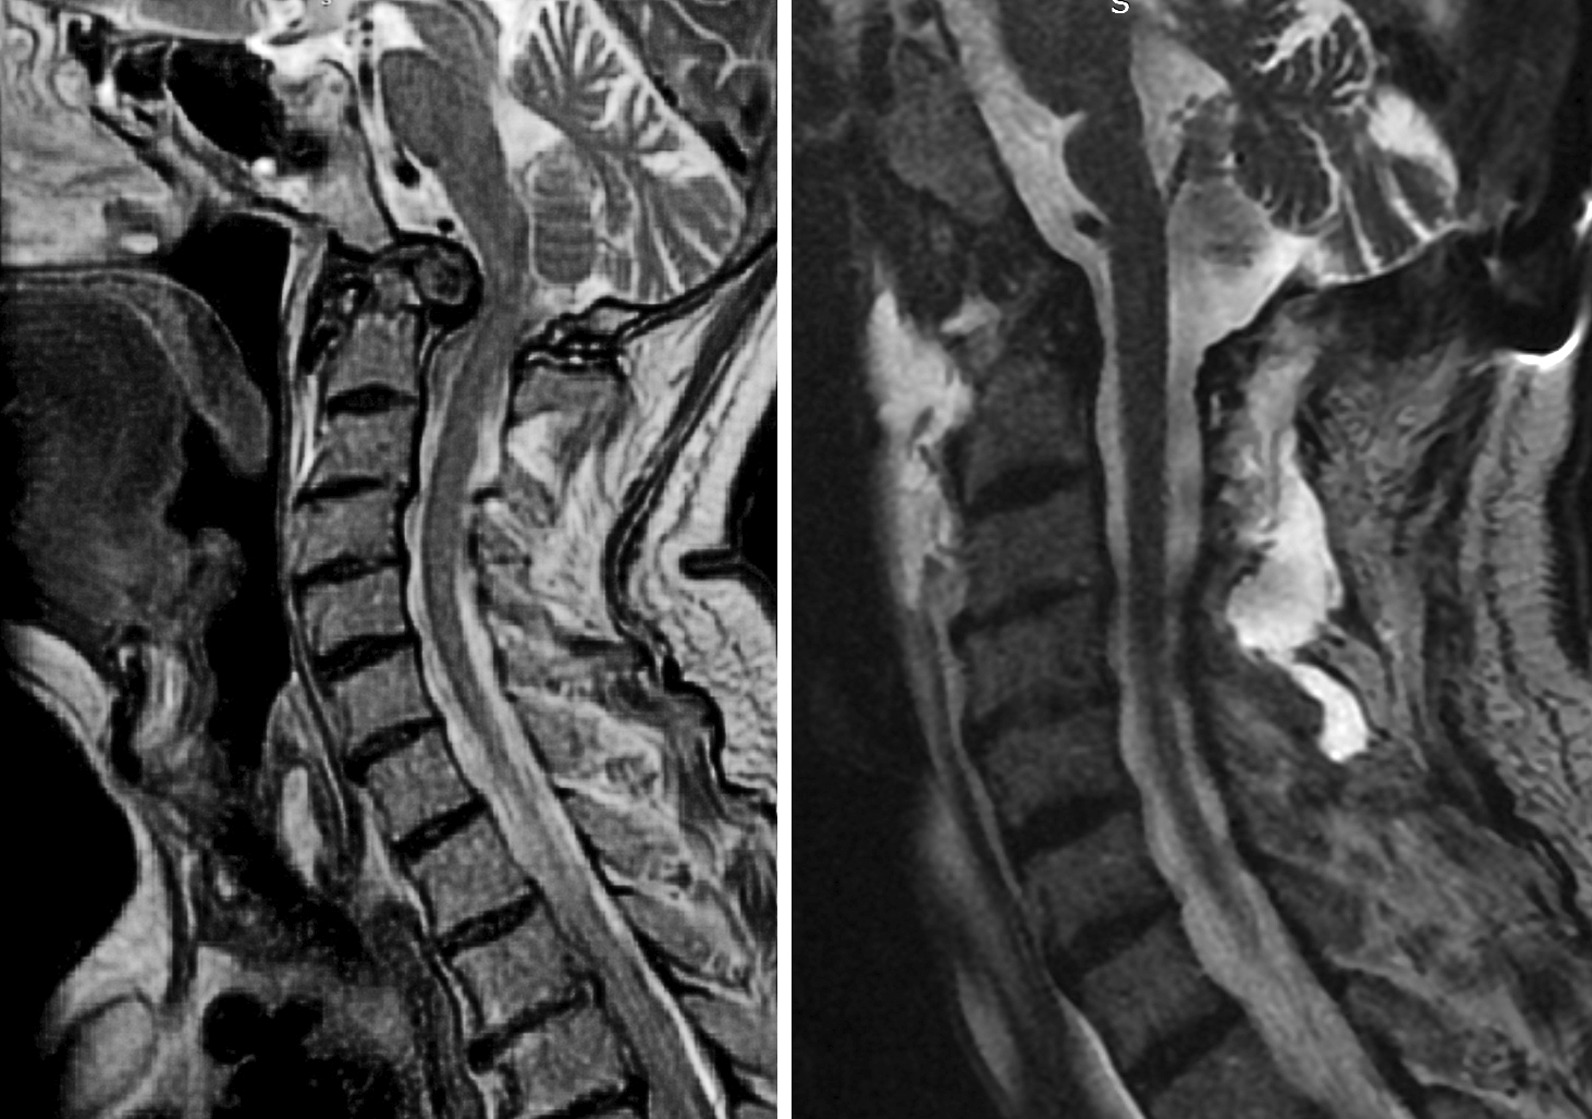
The preoperative (left) and postoperative (right) magnetic resonance (MRI-T2) imaging of the male patient in his 60 s. All images, figures and additional materials are the authors’, no materials are used from another source
